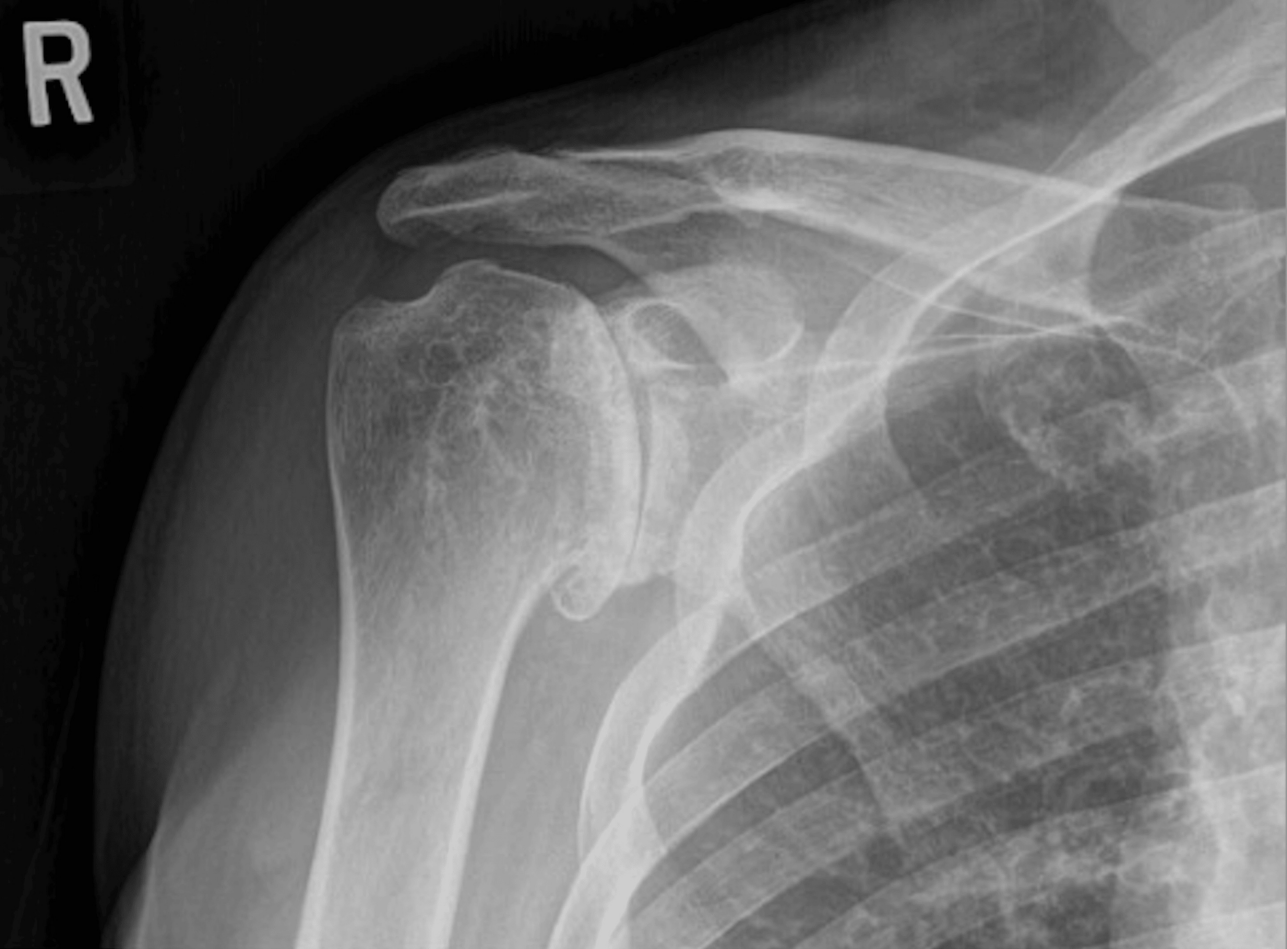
Preoperative X-rays of the right shoulder The image depicts a Grashey view of the right shoulder
